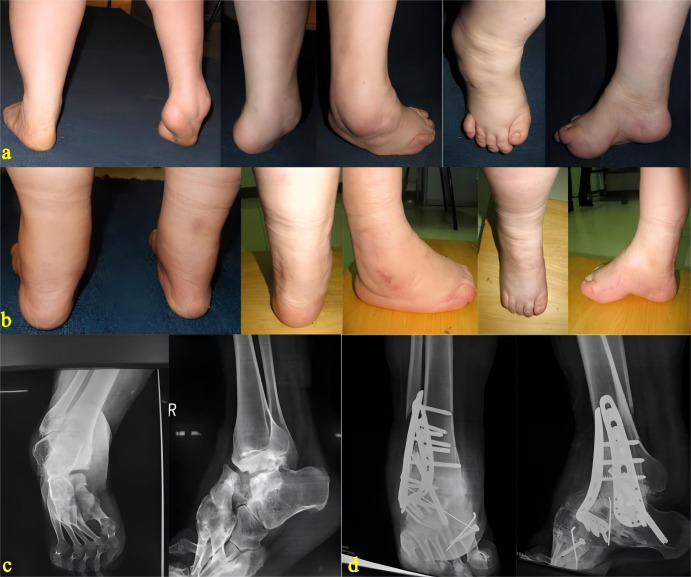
Changes in the patient's appearance and X-ray findings preoperative and postoperative. (a) Preoperative appearance of the foot, showing hindfoot varus, a high arch, and a talipes equinus deformity; (b) Postoperative appearance of the foot, demonstrating significant improvement in hindfoot alignment and the high-arch deformity; (c) Preoperative X-ray, revealing marked degeneration in the midfoot and hindfoot joints, with most foot joints exhibiting malalignment; (d) Postoperative X-ray, showing extensive joint corrective fusion performed, with the deformity well corrected.
